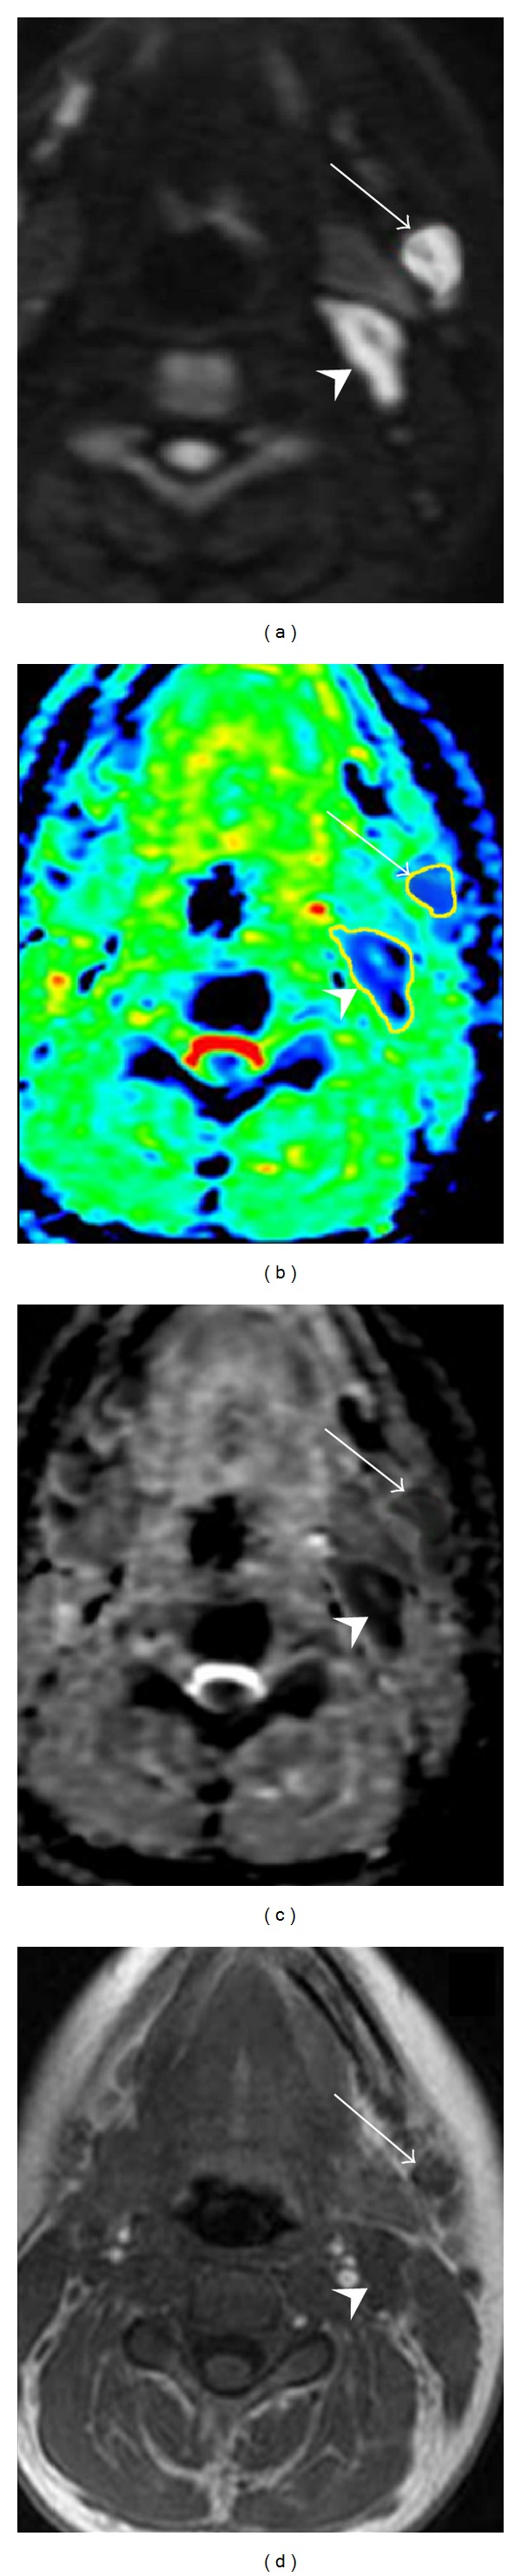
Axial native DWI image at b value of 1000 sec/mm^2^ (a), color ADC map (b), grey scale ADC map (c), and T1-weighted image (d) of a patient with nasopharynx carcinoma showing two solid lymphadenopathies located at level Ib (arrow) and level IIa (head arrow), respectively. According to morphological criteria the lymph node at level Ib, round shaped and 6 mm in size, was considered to be negative for cancer, while the adenopathy localized at level IIa, oval shaped and with a short transverse diameter of 11 mm, was reported to be suspicious for metastatic involvement. Concerning DWI, level Ib lymph node showed a mean ADC value of 0.690 × 10^−3^ mm^2^/sec and was considered suspicious for metastatic involvement, whereas level IIa adenopathy displayed an average ADC value of 1.051 × 10^−3^ mm^2^/sec and, therefore, was deemed to be a noncancerous lymphadenopathy. At pathological examination level Ib lymph node showed large intranodal metastatic deposits and level IIa adenopathy was found to be benign.
